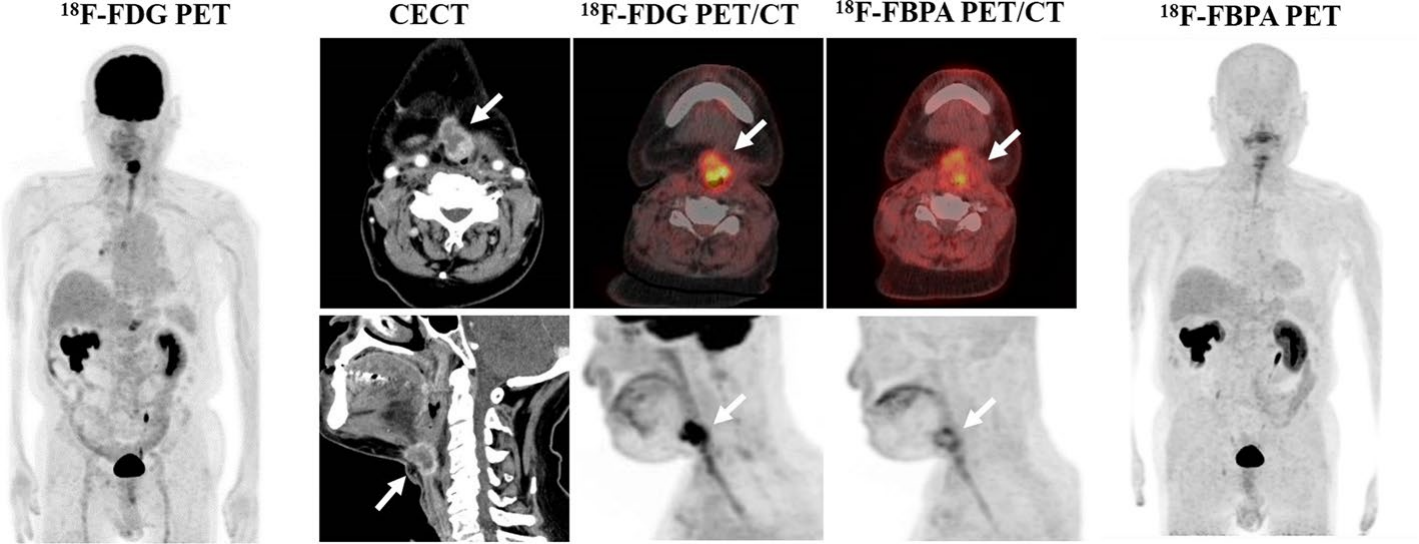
A 75-year-old man underwent contrast-enhanced CT to confirm local recurrence of hypopharyngeal cancer after radiotherapy. Clinically, post-treatment changes (granulation tissue, radiation necrosis) were suspected, but both ^18^F-FDG (SUVmax: 13.0) and ^18^F-FBPA (SUVmax: 5.8) PET/CT showed increased accumulation. Local recurrence was confirmed pathologically by puncture cytology
